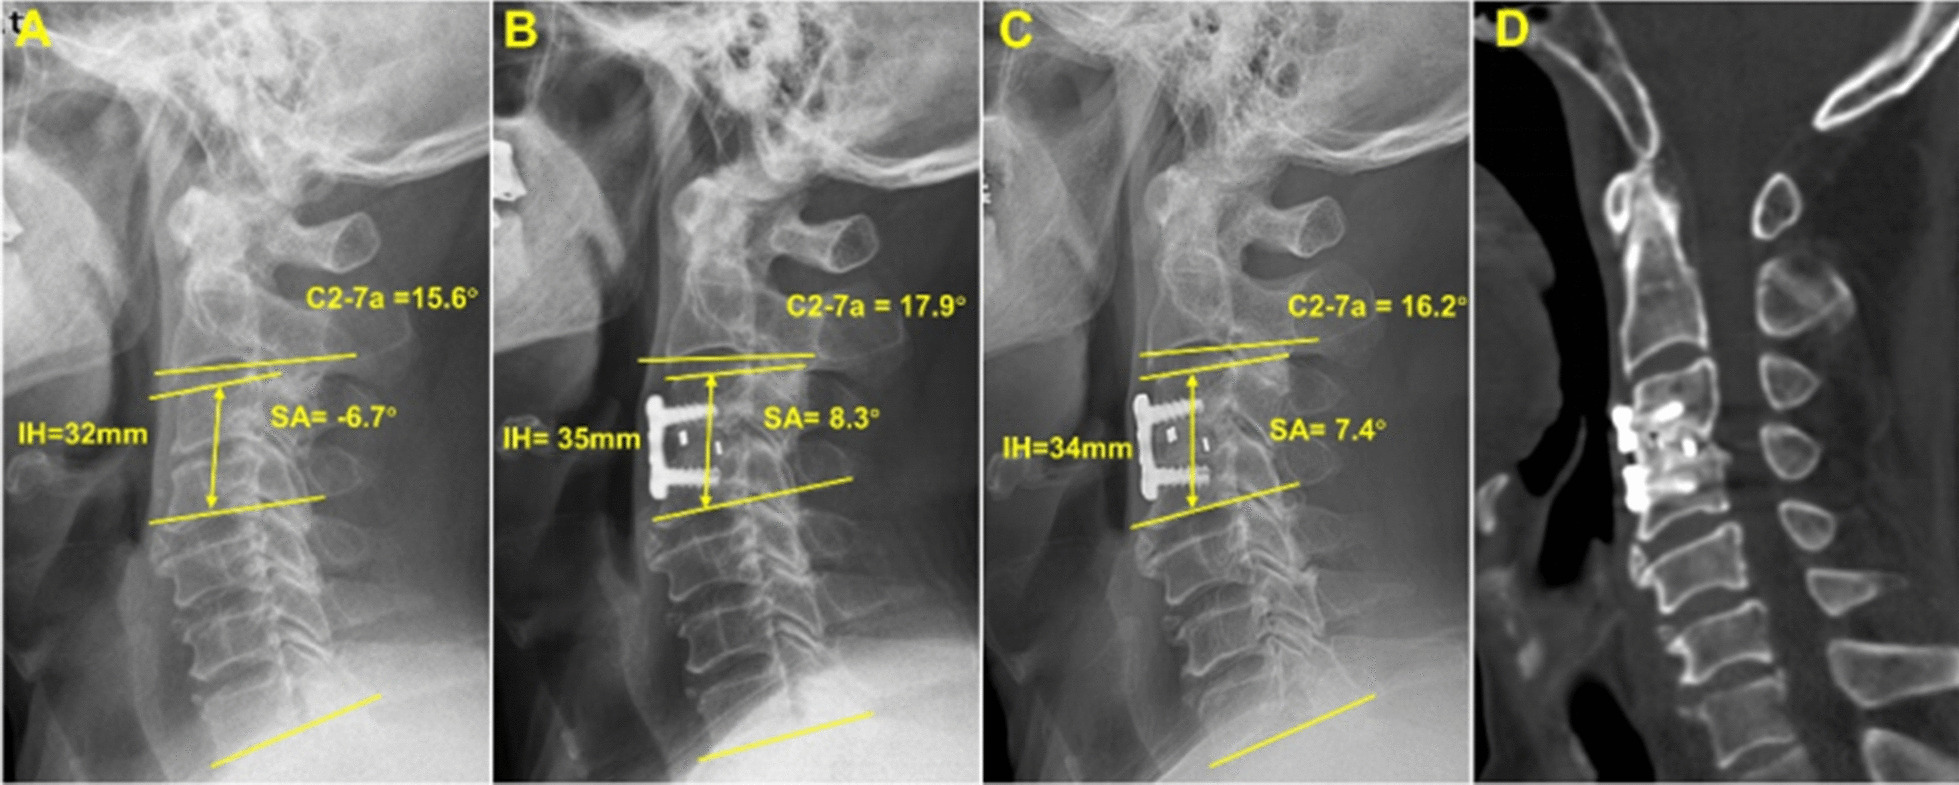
A 67-year-old man underwent ACDF with a novel n-HA/PA66 cage. a Preoperative lateral radiograph. b Lateral radiograph at 6-month post-surgery. c Lateral radiograph at the final follow-up. d 3d-CT at the final follow-up
